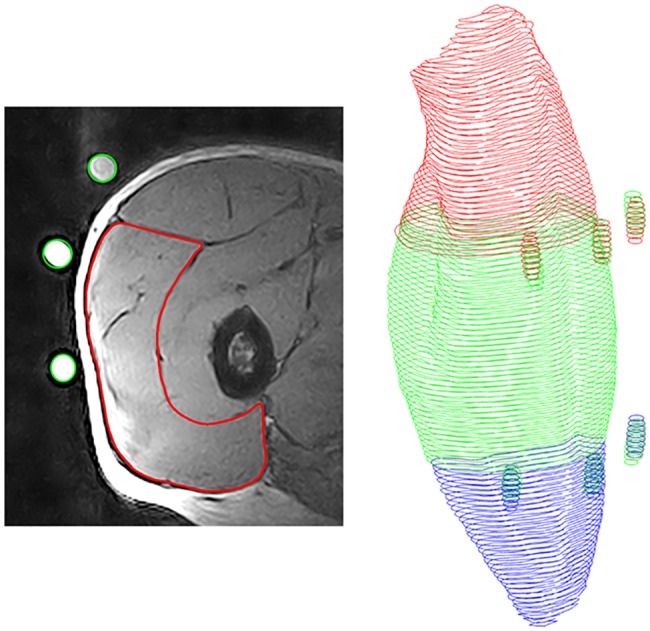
Procedure of muscle reconstruction. Left: Magnetic resonance image of the vastus lateralis with segmented muscle (red) and identified fish oil capsules (green); Right: Reconstructed muscle with fish oil capsules, proximal part (red), medial part (green) and distal part (blue).
